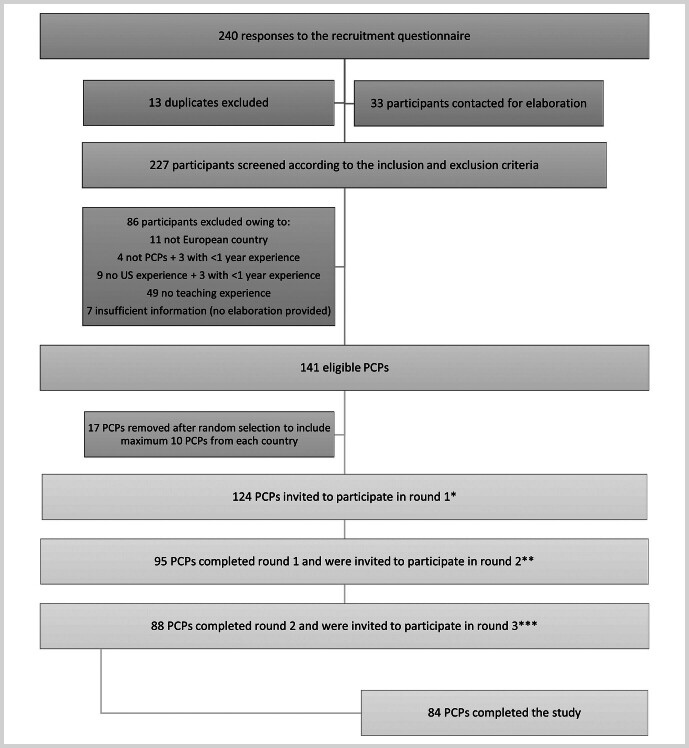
Recruiting the Delphi panel. PCP = primary care physicians, US = ultrasound * Survey round 1 was distributed on Dec 18
